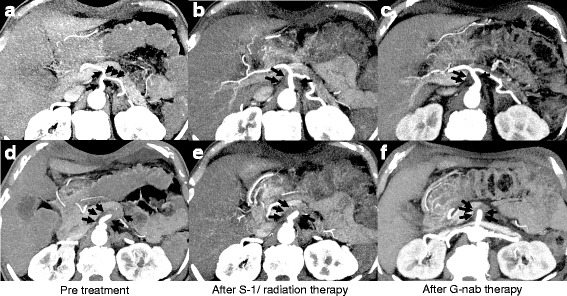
Axial computed tomography images showing the celiac artery, common hepatic artery, splenic artery, and superior mesenteric artery. a, d Before chemoradiation therapy. b, e After chemoradiation therapy. c
